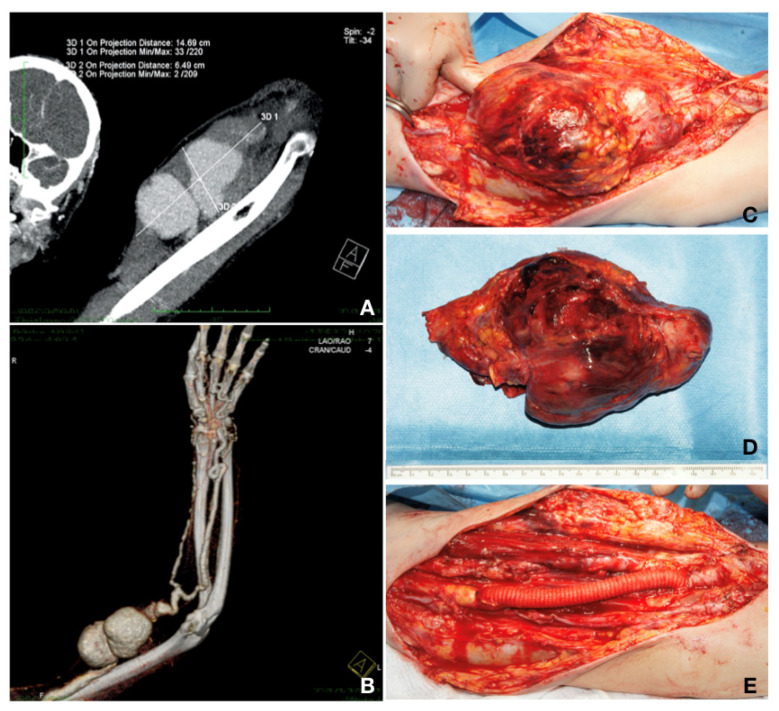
(A,B) MIP thin collection and volume rendering technique (VRT) CT-Angio showing the 2D dimensions of the giant aneurysm. On the VRT image, one can observe the bad quality of the radial artery and the calcified aspect of both run-off vessels distal to the aneurysm. (C) Intraoperative image with the aneurysm dissection. (D) Resected aneurysm with mural thrombosis. (E) Vascular reconstruction with 10 mm Dacron graft interposition and spatulated, end-to-end anastomosis with Prolene 6.0 continuous sutures (personal collection).
